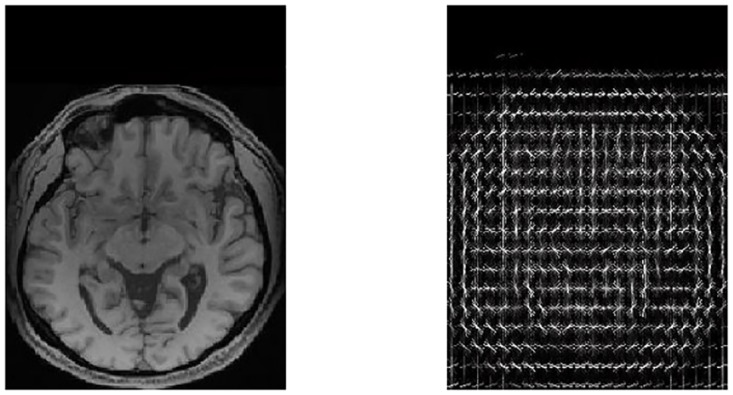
Input and output of 2D HOG on a brain image. The left panel shows an axial slice of a structural MR image of a brain. The right panel shows the HOG features of the same slice. Here, we represent the HOG features by an 8-sided “star”, where the length of each arm is the size of the histogram in that direction. This representation is generated using VLFeat [32].
